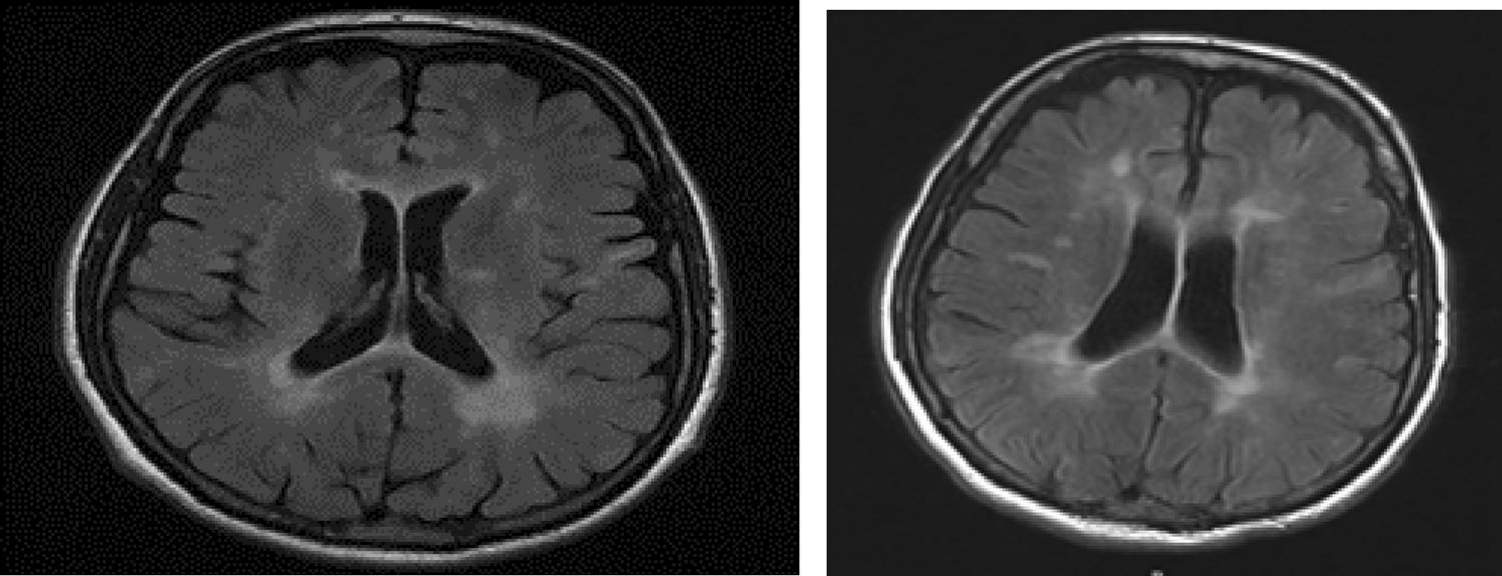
MRI brain showing T2 and FLAIR Abnormal signal hyperintensity in deep white matter tracts cerebral parenchyma—case 1
